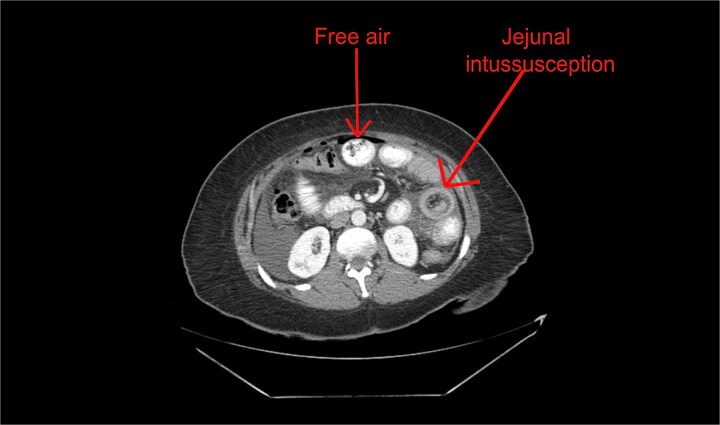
Contrast-enhanced CT demonstrating mesenteric swirl sign (arrow), venous congestion, and free intraperitoneal air consistent with bowel perforation.
